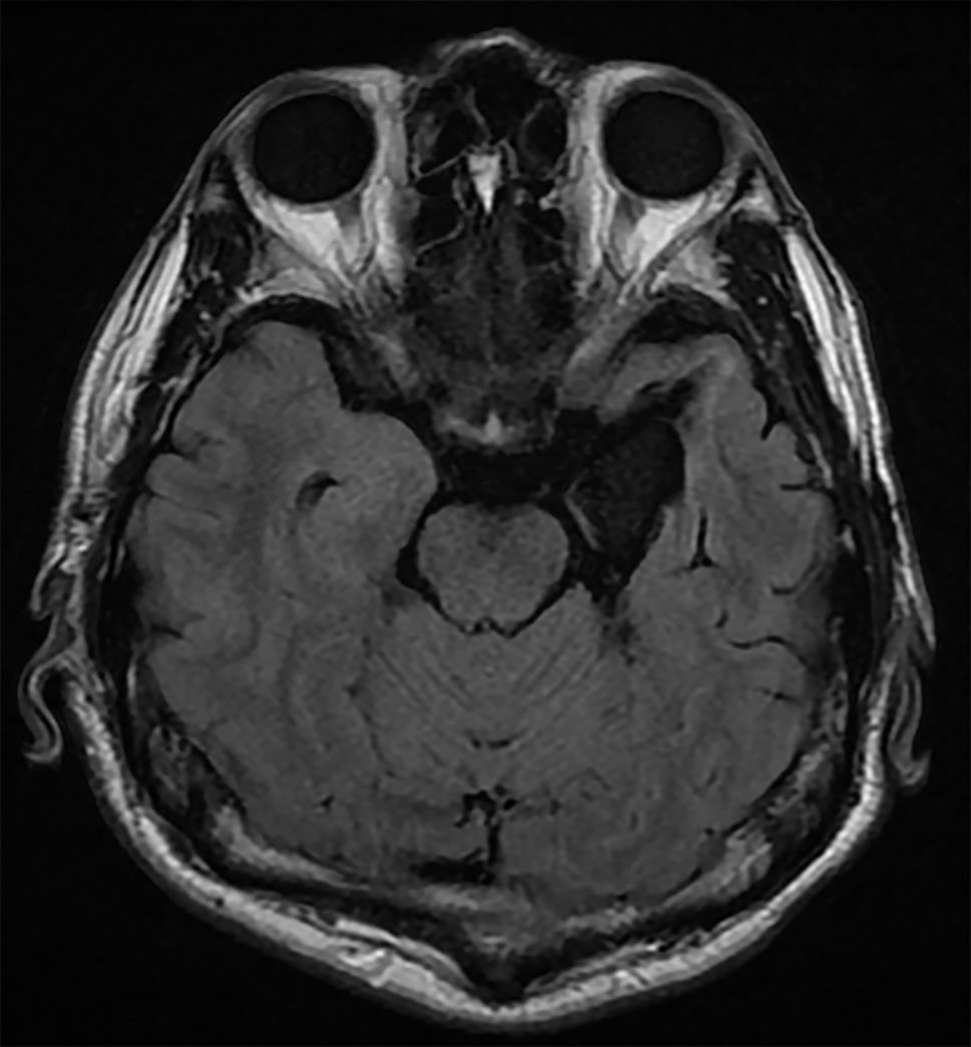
Representative anatomical magnetic resonance image of a patient after a medial temporal structure resection.
